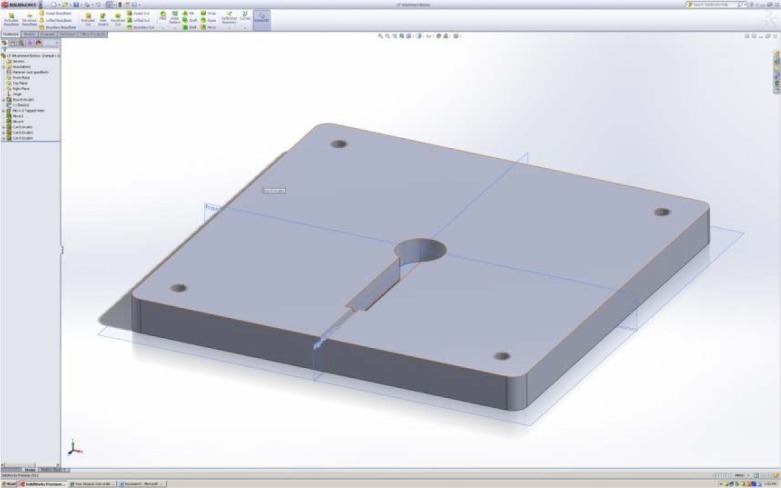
Lower build plate that houses ultrasonic transducer.
